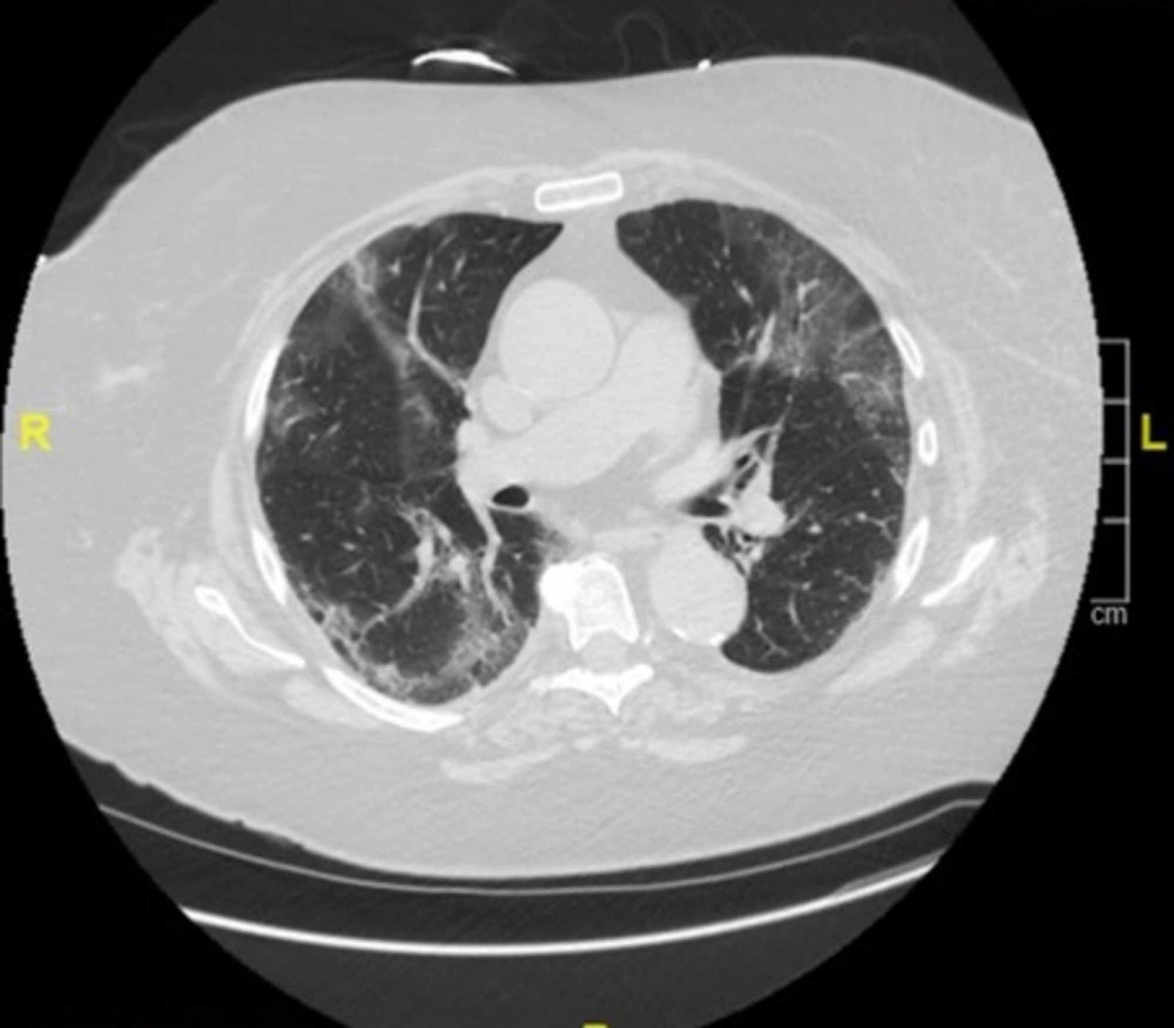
CT scan showing ground-glass capacities in the lung peripheries CT scan of the patient at the time of admission shows bilateral, ill-defined, ground-glass infiltrates.
